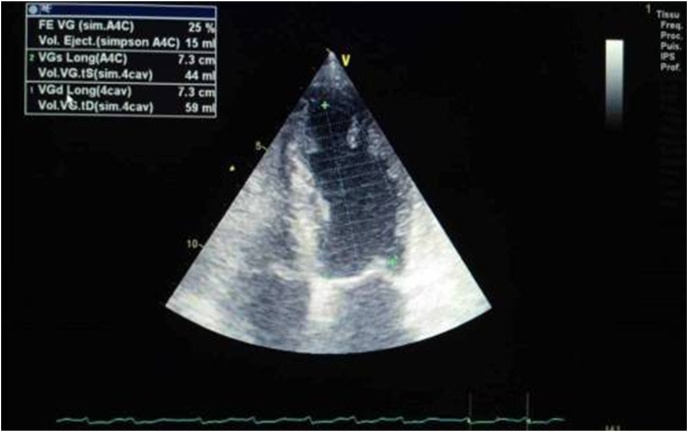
Transthoracic echocardiography showing severe systolic dysfunction at 25%.
